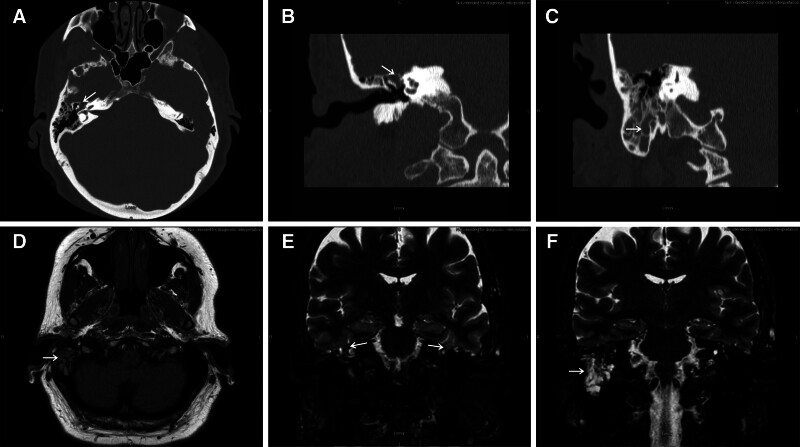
Bilateral temporal encephaloceles. Computed tomography (CT) (*A*–*C*) and MRI (*D*–*F*) images of a patient with bilateral temporal encephaloceles and right mastoid effusion. *A*, Axial CT showing right encephalocele between the cochlea and ossicular chain (arrow). *B*, Coronal CT showing right encephalocele between the cochlea and ossicular chain (arrow). *C*, Coronal CT posterior to the previous image showing mastoid effusion (arrow). *D*, Axial T-1 weighted MRI image showing right mastoid effusion (arrow). *E*, Coronal T-2 weighted MRI showing bilateral temporal encephaloceles (arrows). *F*, Coronal T-2 weighted MRI image showing right middle ear effusion (arrow).
